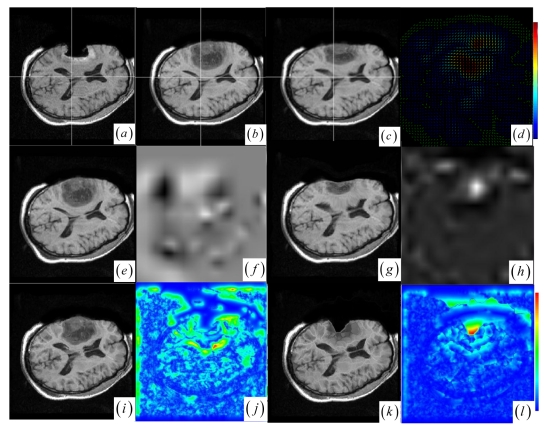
(a)–(b) Intra- and pre-operative MR images. (c) JKC. (d) Displacement vector field. (e) BMI. (f) BMI deformation image. (g) BCR. (h) BCR deformation image. (i) DEM. (j) DEM deformation image. (k) DIF. (l) DIF deformation image.
